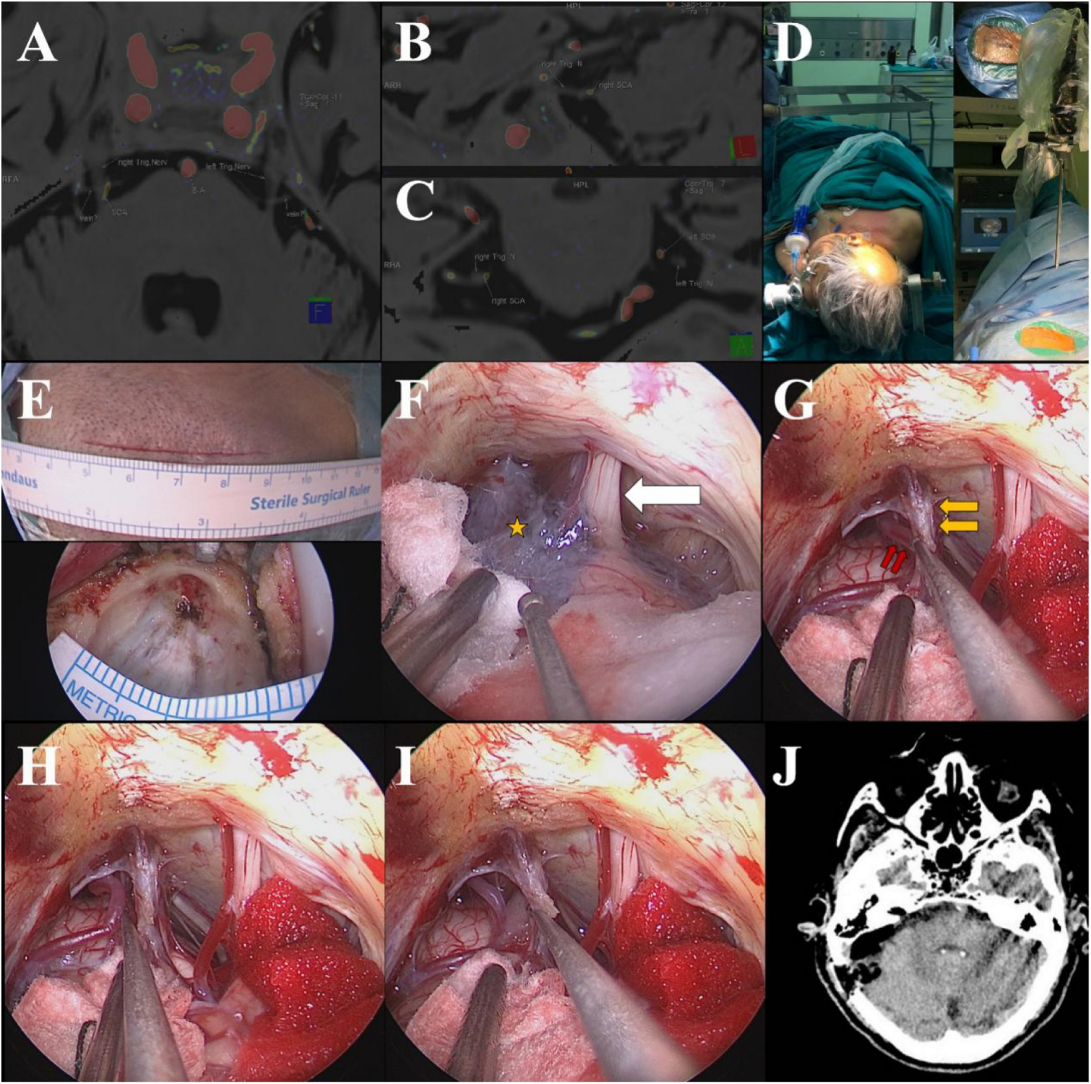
MRTA clearly showed the relationship between the right REZ of the trigeminal nerve and the right SCA (A–C); supine position after the general anesthesia and the neuroendoscope fixed with a pneumatic arm (D); the incision [(E), upper] and craniectomy [(E), lower]; endoscopic visions showed the thickening of the arachnoid membrane (yellow star) in the cistern of CPA and the cranial nerve VII/VIII (white arrow) (F); the right SCA (the double red arrows) and the right trigeminal nerve in front of the right SPV (double yellow arrows) (G); the right SCA being dissected away from the right REZ of the trigeminal nerve (H); Teflon spacer being interposed between the REZ and the SCA (I); head CT scan immediately after the operation (J).
